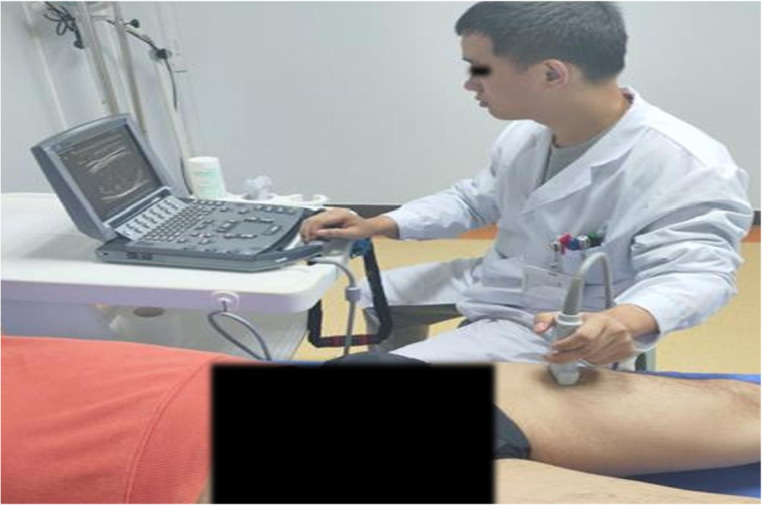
MSK ultrasound and its application.
